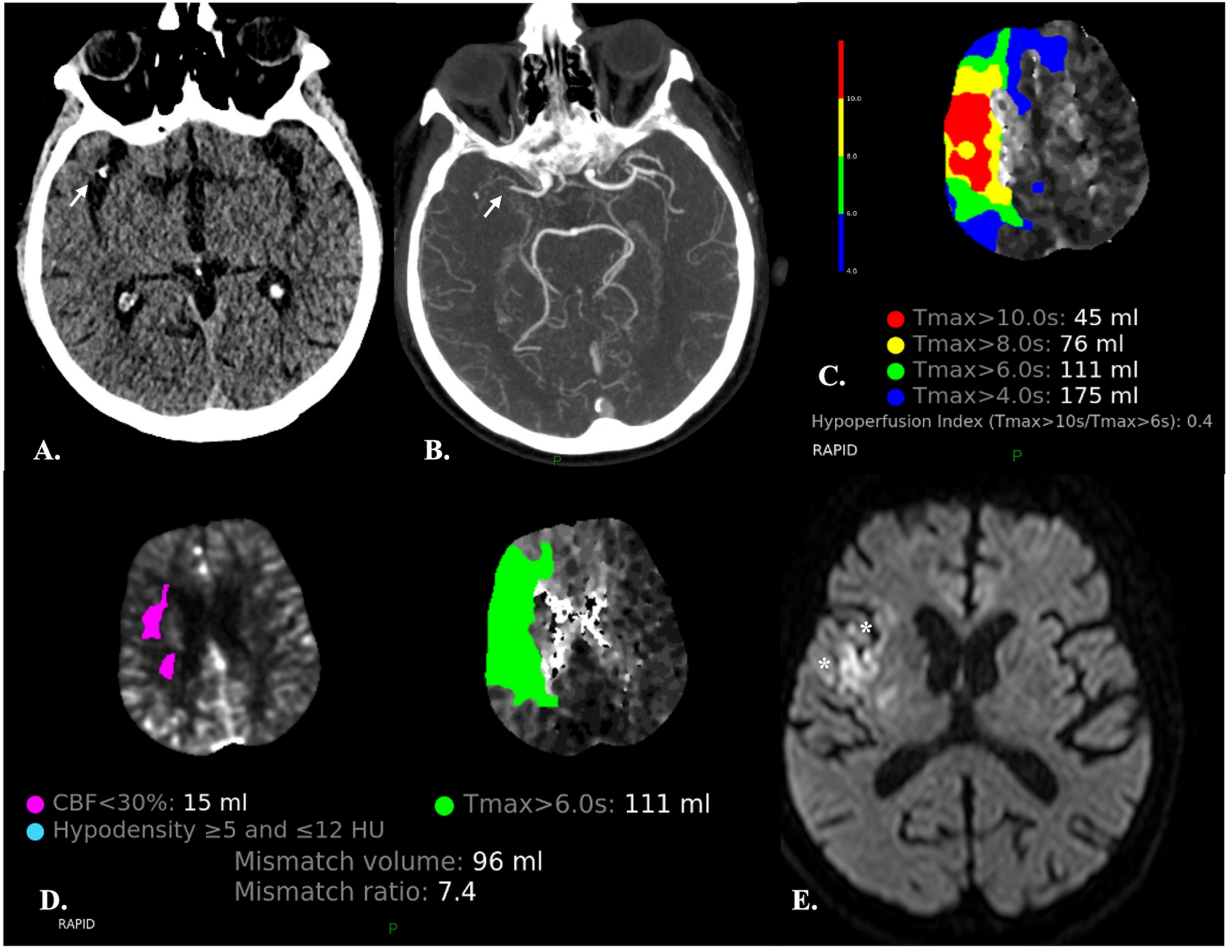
Automatic segmentation of the brain parenchyma into normal tissue, tissue at risk (TAR) and non-viable tissue (NVT) by the CTP RAPID software in a 67-year-old male with abrupt onset left-sided hemiparesis. (A,B) NECT (A) and CTA (B) demonstrate occlusion of the M1 segment of the right MCA (*arrows)* and hypodensity of the insular cortex (not shown) resulting in an ASPECTS score of 9. (C) The time-to-maximum (TMax) CTP colour map shows different TMax thresholds (10, 8, 6 and 4 s) for TAR estimation: the lower the threshold, the larger the TAR volume. The hypoperfusion intensity ratio (HIR) (i.e., the Tmax > 10 s/Tmax >6 s ratio) is also provided. A low HIR is considered a predictor of slow ischemic core growth. Conversely, a high HIR is associated with a rapid growth of the infarct core (44). (D) Automated quantification of TAR and NVT and calculation of mismatch ratios are provided for treatment decision. Automated identification of areas with density varying between ≥5 and ≤12 HU, presumably consistent with acute ischemic lesions, is also carried out. (E) Diffusion-weighted MRI obtained two days after mechanical thrombectomy showed a very limited area of ischemia ()* as compared to the previously observed Tmax > 6 s, indicating that reperfusion has been effective in salvaging the ischemic penumbra.
